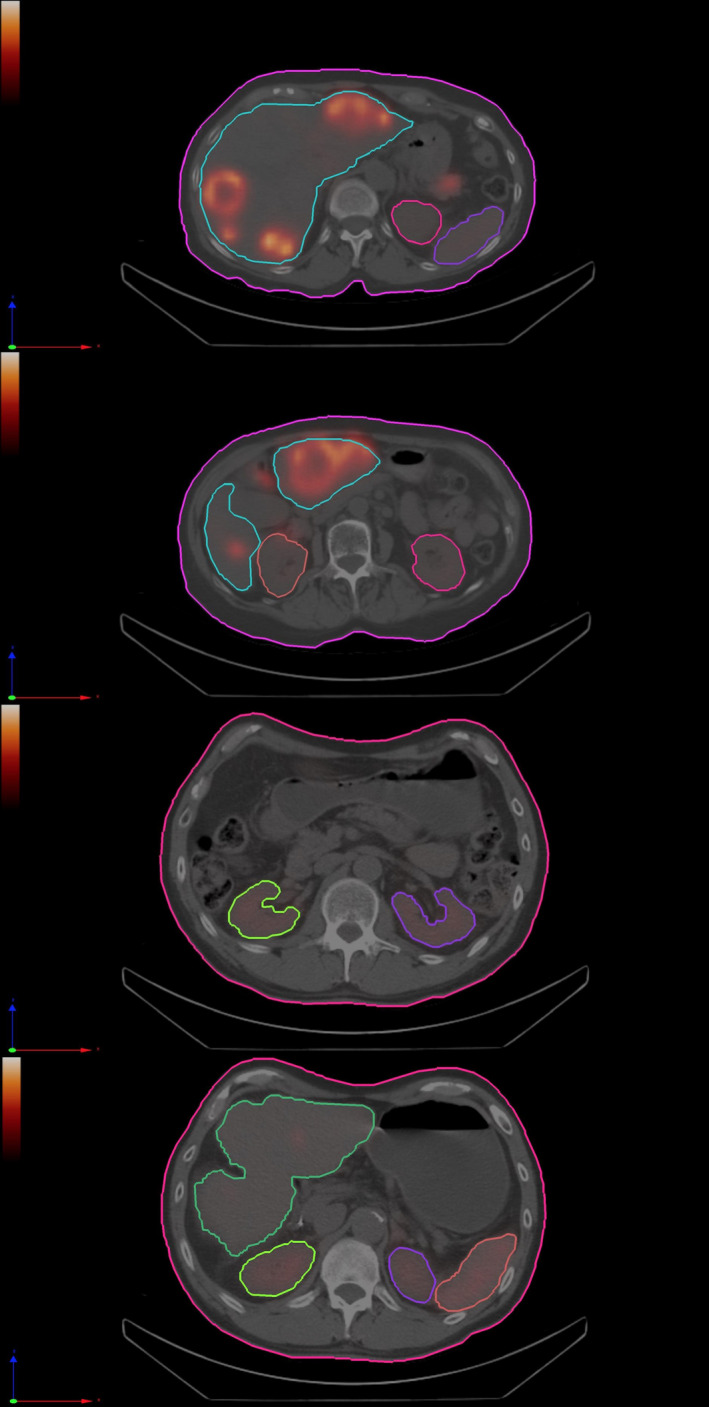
Two different axial slices showing segmented organs (liver, spleen, and kidneys) in the reference computed tomography, using PDOSE workstation (image reconstruction was performed using HROD). (a), (b) female patient first treatment cycle; (c), (d) male patient first treatment cycle. [Color figure can be viewed at wileyonlinelibrary.com]
